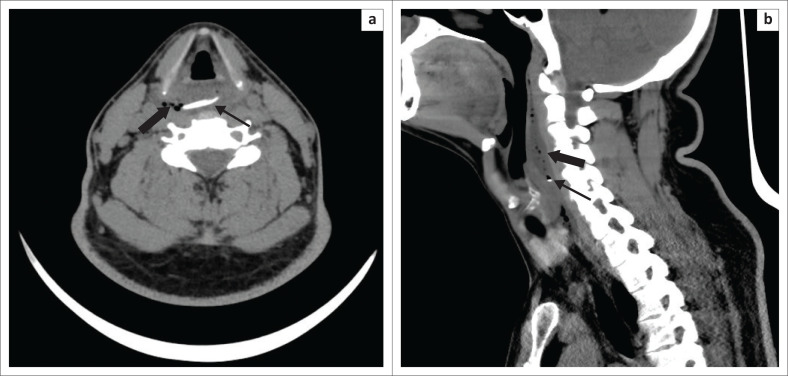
Axial (a) and sagittal reformatted (b) computed tomography images showing a 4 cm × 1 cm fish bone (thin arrows) impacted in the posterior cricopharyngeal wall with perforation resulting in a retropharyngeal abscess (thick arrow).
